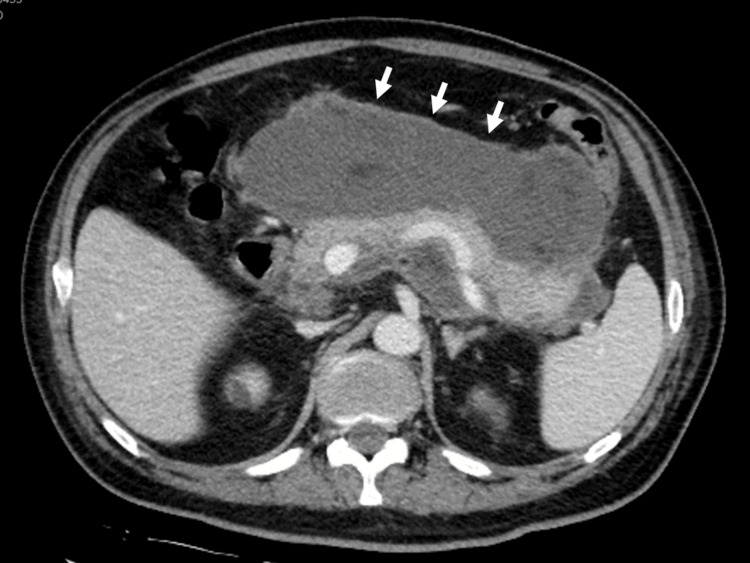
Abdominal CT scans at diagnosis of WON CT scans of the abdomen show a heterogenous collection showing liquid and non-liquid components with a well-defined wall around the pancreas (white arrow) CT: computed tomography, WON: walled-off necrosis
